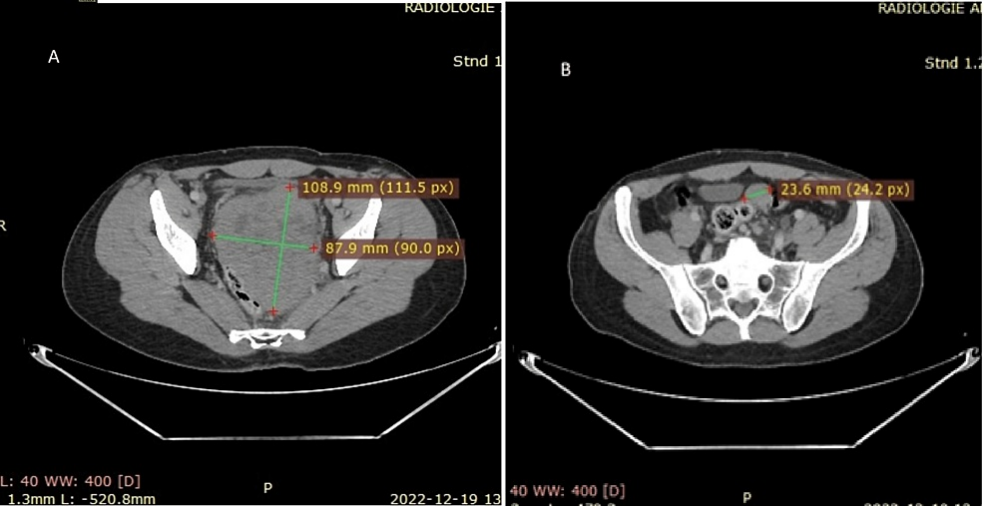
Thoraco-abdominal-pelvic CT scan images A: Large pelvic mass developed at the expense of the prostate; B: Peritoneal nodule
